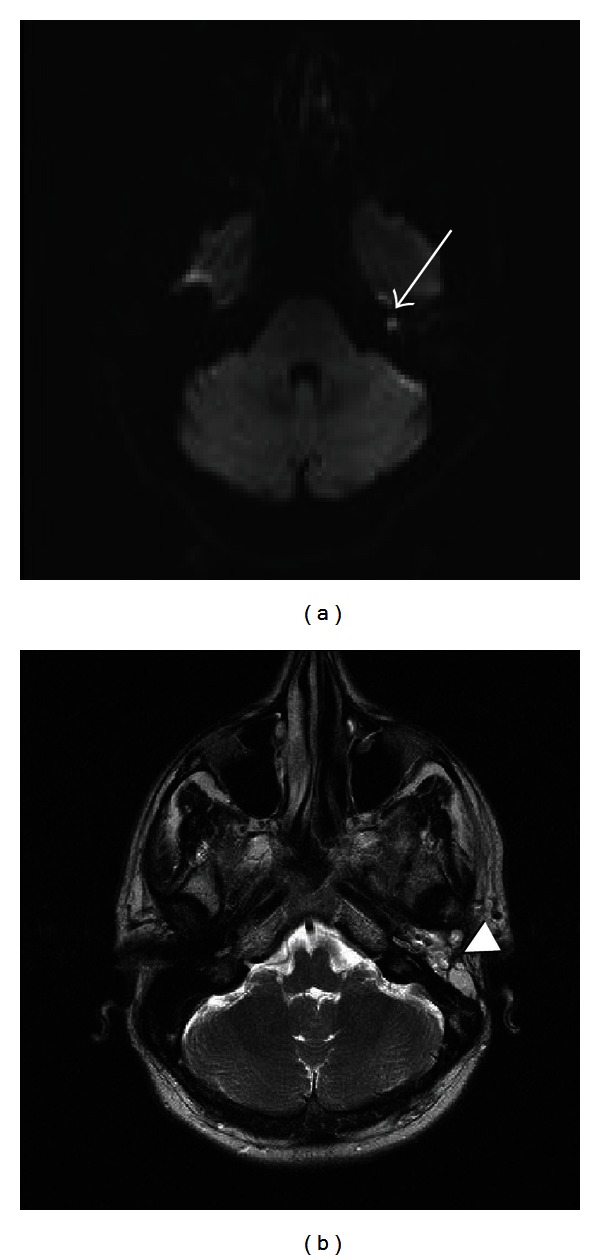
Recurrent cholesteatoma. Axial diffusion-weighted image (a) demonstrates abnormal hyperintense signal in the left temporal bone consistent with a focus on recurrent cholesteatoma (white arrow). Axial T2-weighted sequence (b) shows fat packing material in the left temporal bone (arrowhead) following mastoidectomy with blind sac closure.
